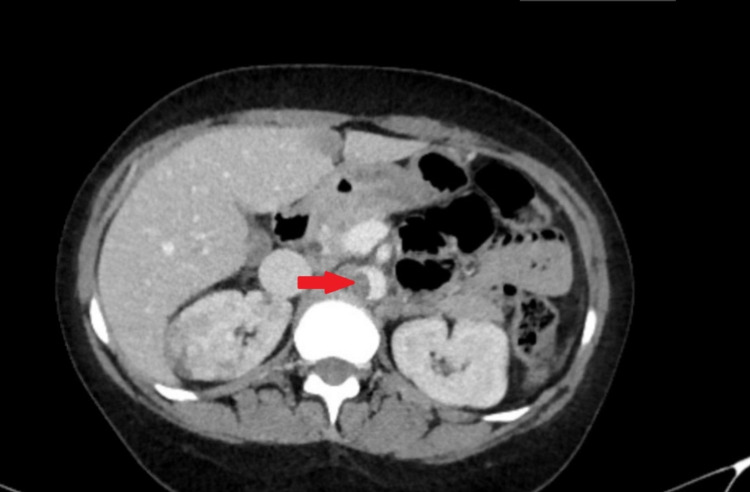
CT abdomen showing thrombus along the wall of abdominal aorta.
